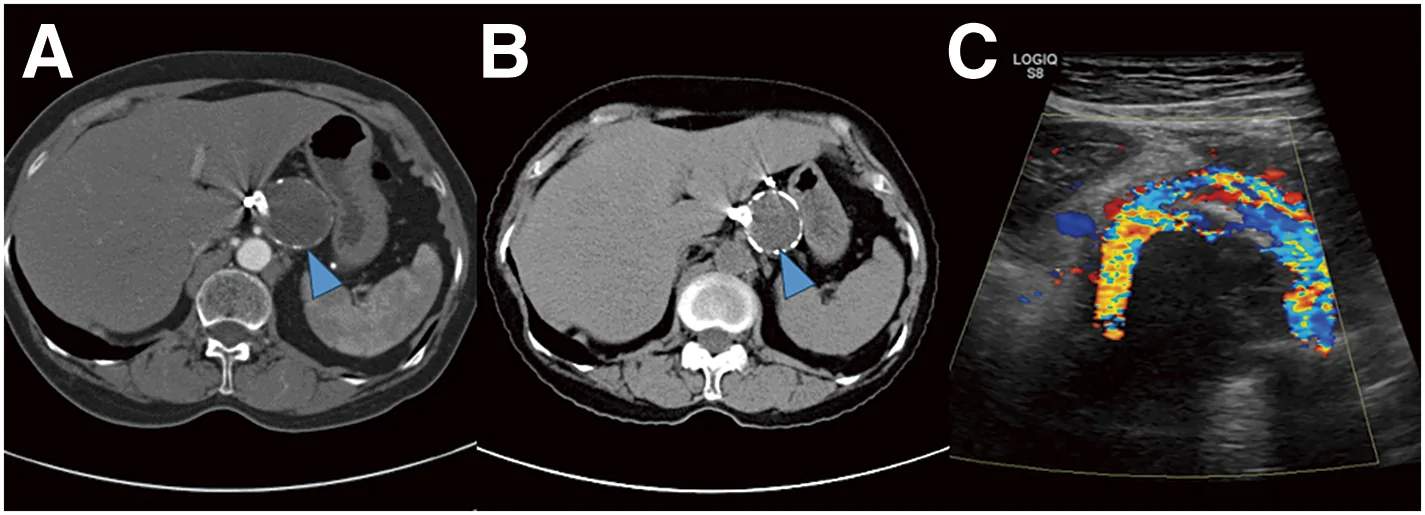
Follow-up images. (A) Postoperative CT showed no endoleak within the aneurysm (arrowhead). (B) CT obtained 13 years after EVT showed aneurysm shrinkage (arrowhead). (C) DUS obtained 13 years after EVT demonstrated patency of the covered stent. DUS, duplex ultrasound; EVT, endovascular treatment
